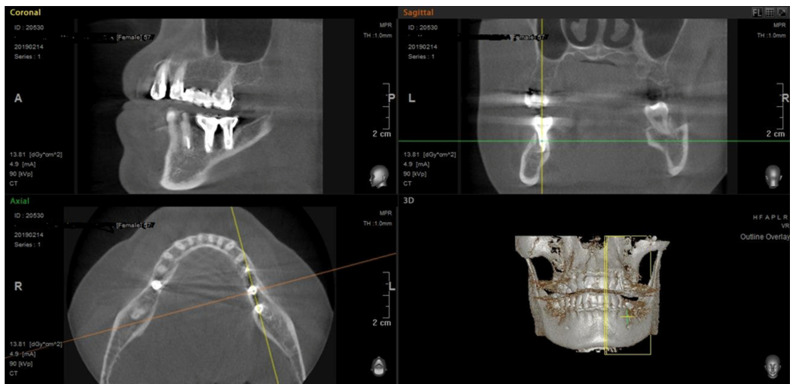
Patient’s CBCT example 6 years after operation. The D2-type bone density around dental implants has been shown in the presence of both types of bone (cortical and sponge) and the absence of pathological bone resorption signs (zone of interest is marked with cross of yellow and red lines).
